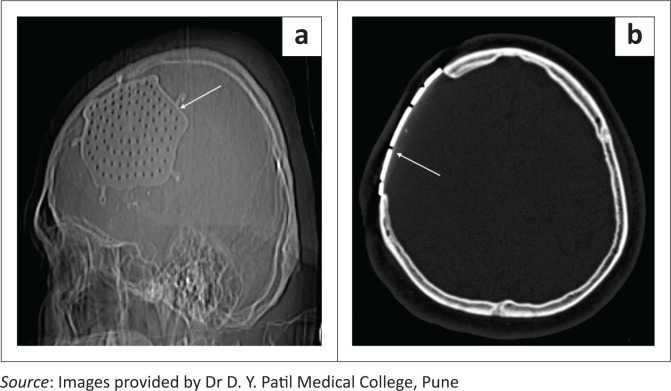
(a, b) CT scanogram and axial bone window CT image demonstrating a right fronto-parietal craniectomy with a titanium cranioplasty implant (long white arrows).
